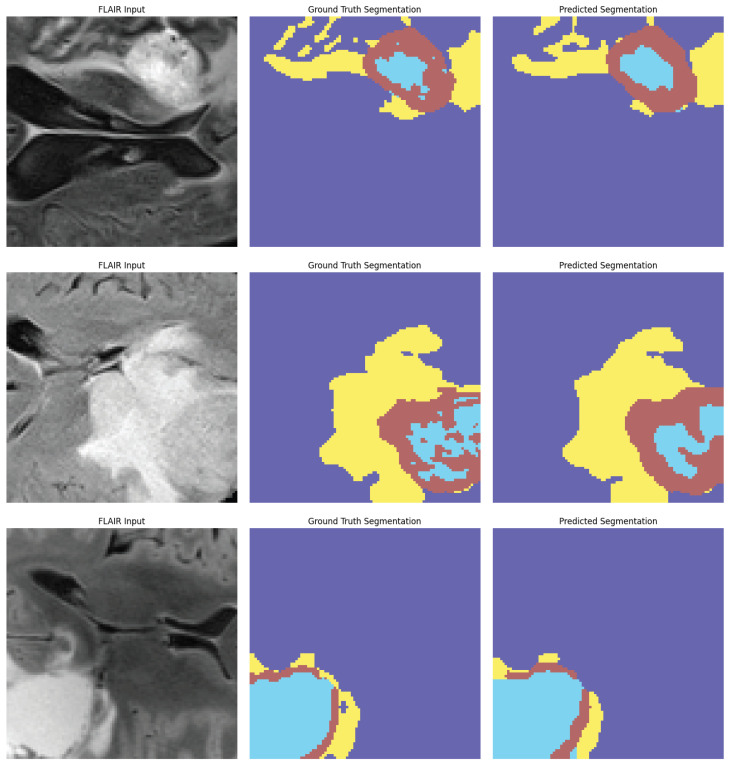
Qualitative results. Representative FLAIR slices with ground-truth masks and AVLT predictions; salient tumor subregions are captured consistently with the reference annotations.
